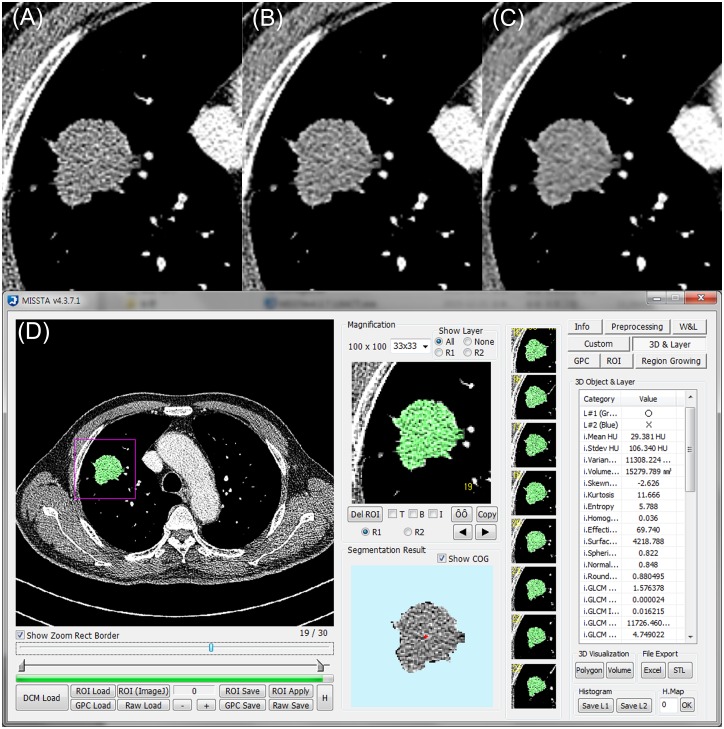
A pulmonary mass (nasopharyngeal carcinoma metastasis) in a 59-year-old male. CT images were reconstructed with (A) filtered back projection and Sinogram Affirmed Iterative Reconstruction (B) level 3 and (C) level 5. (D) Tumor was segmented manually and radiomic features were automatically calculated using in-house software.
